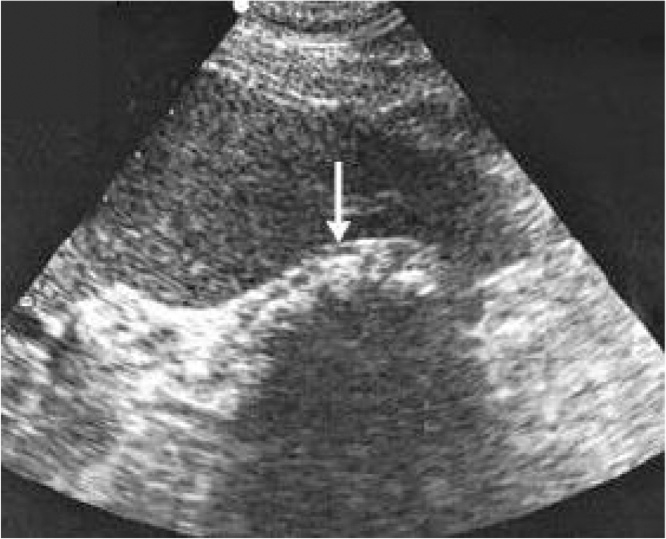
Ultrasound shows a constricted gallbladder with the possible acoustic shadow in its projection.
